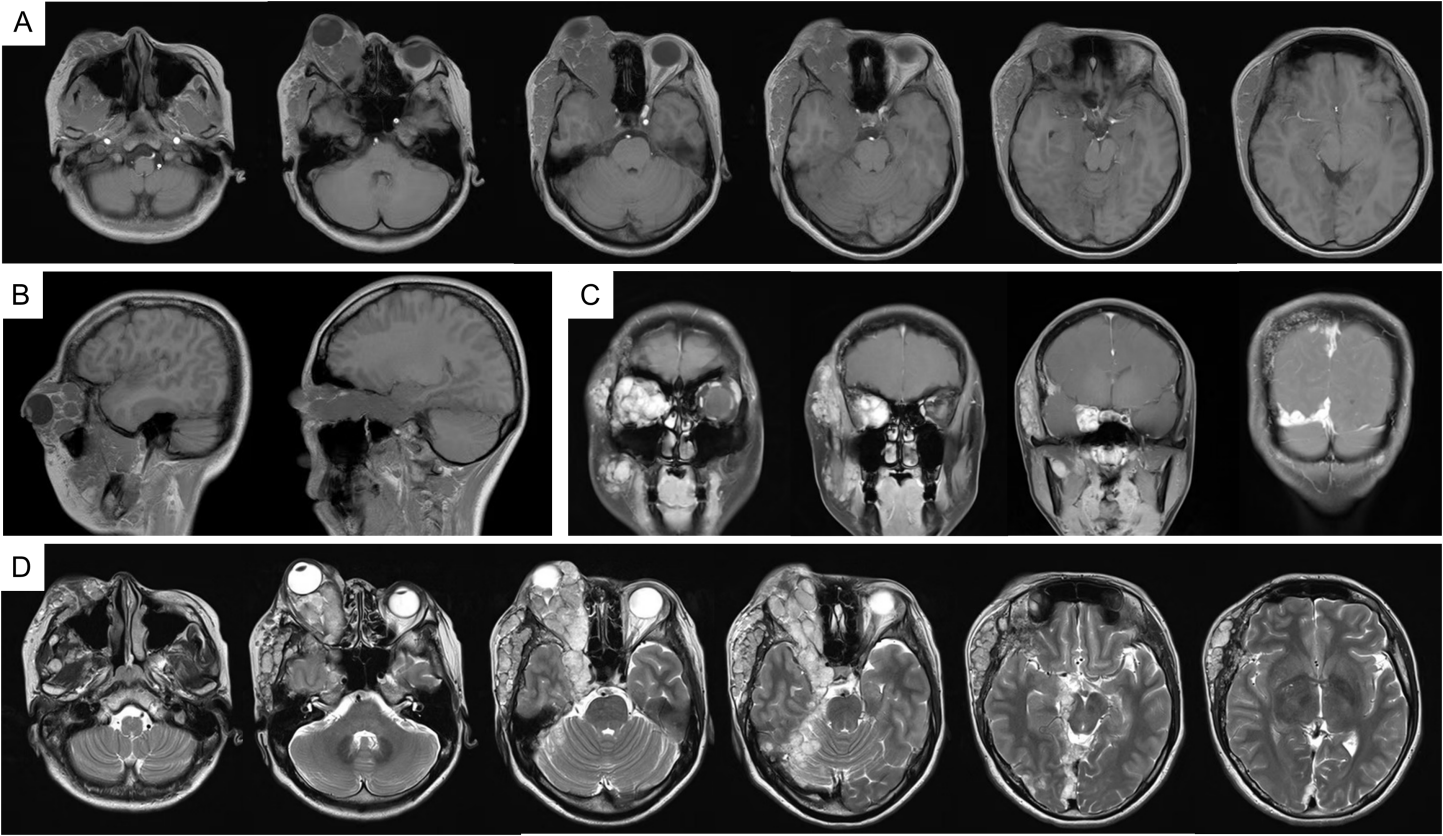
MRI imaging in 2023. (A) T1WI; (B) T1WI-C coronal view; (C) T1WI-C sagittal view; (D) T2WI-TSE. MRI, magnetic resonance imaging; T1WI, T1-weighted image; T2WI, T2-weighted image; T1WI-C, T1WI with contrast; T2-FLAIR, T2-fluid attenuated inversion recovery.
